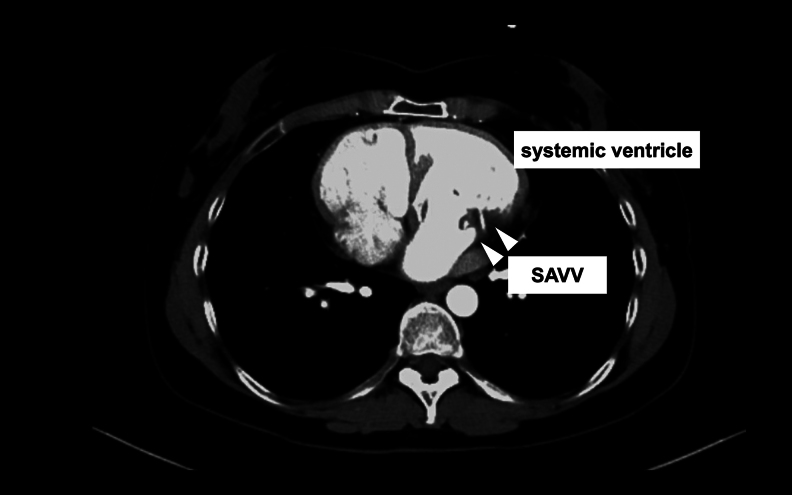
Contrast-enhanced computed tomography showed that systemic atrioventricular valve was vertically oriented (white arrow).
